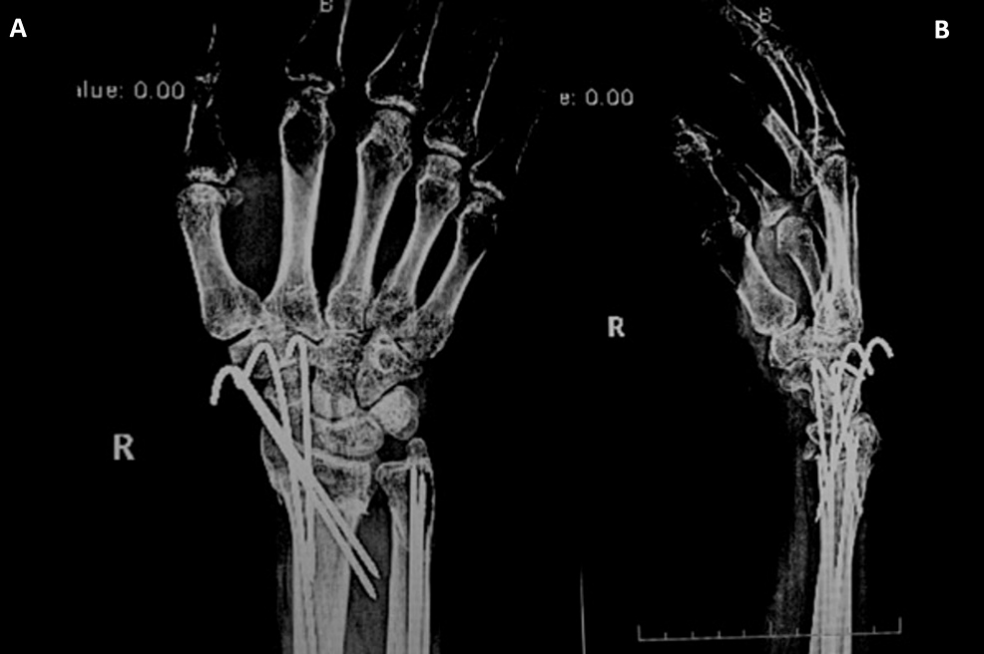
Anteroposterior (A) and lateral (B) wrist and distal forearm radiography done six weeks postoperatively. Out of splint radiographs showing excellent healing with callus formation and alignment maintained (radial height, volar inclination).
